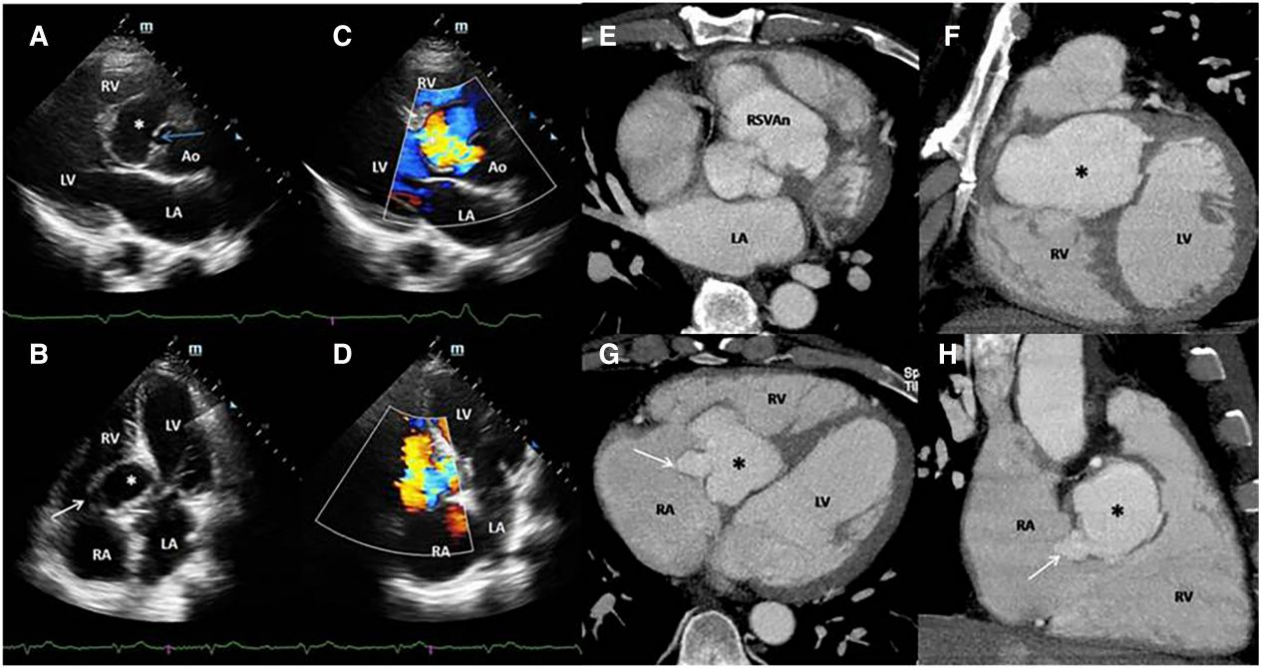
SOVA is a rare but well-known cardiac anomaly. Symptoms typically arise when SOVA ruptures or compresses adjacent structures, leading to heart failure, aortic regurgitation, conduction disorders. In sub-Saharan Africa, rupture into the IVS is the most common complication, often associated with AVB. Echocardiography is the initial imaging modality for suspected SOVA rupture, while CT provides detailed anatomical delineation, especially in cases with multiple ruptures or extensive IVS dissection. Simultaneous rupture of the SOVA into the IVS and left, right or both ventricles is a sporadic heart condition, with 22 cases described in the literature. Concomitant rupture into SIV and RA is even rarer, with only one case described.
